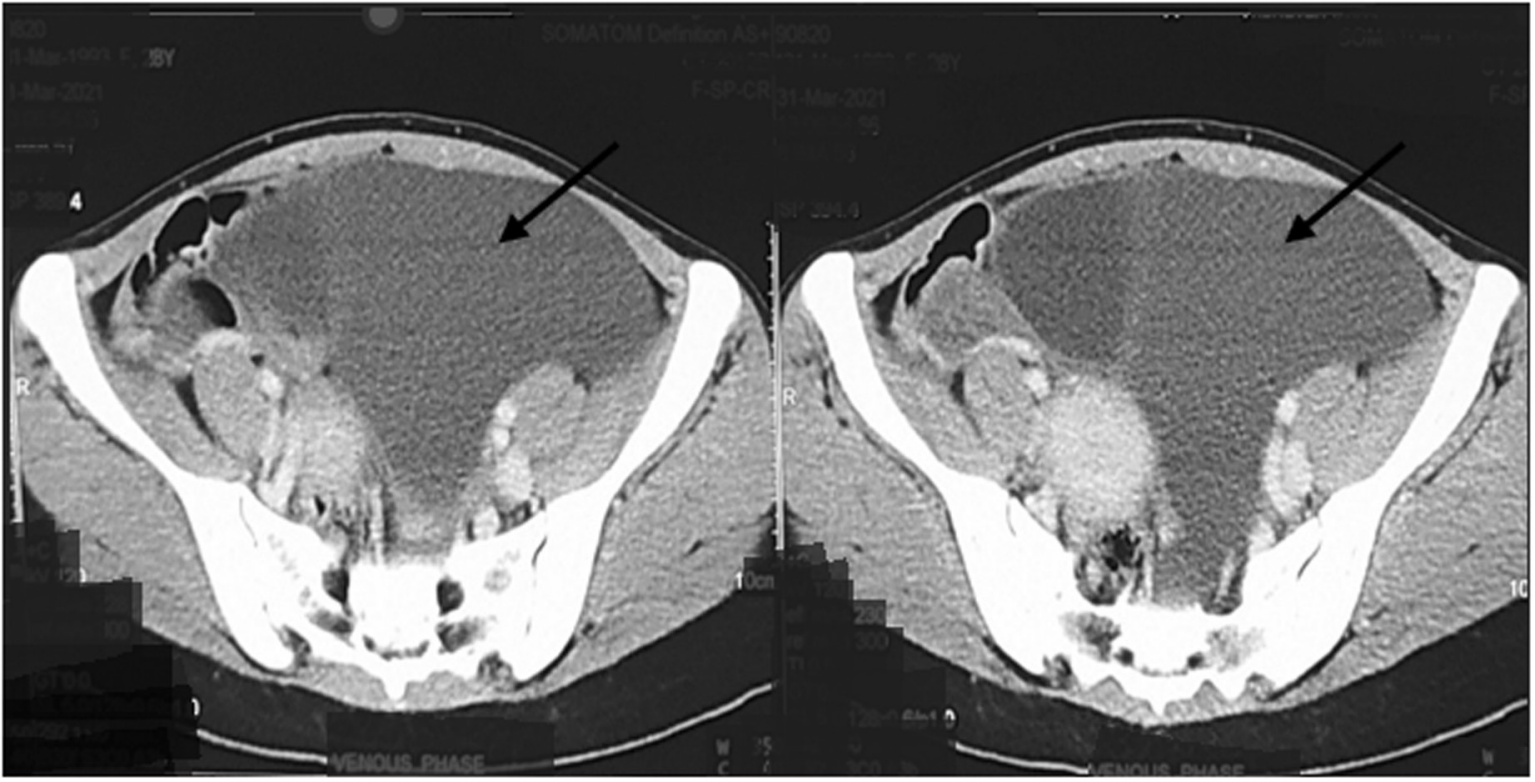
Axial image of contrast‐enhanced computed tomography (CECT) of abdomen/pelvis shows non‐enhancing abdomino‐pelvic cystic mass with no solid component (black arrows)
